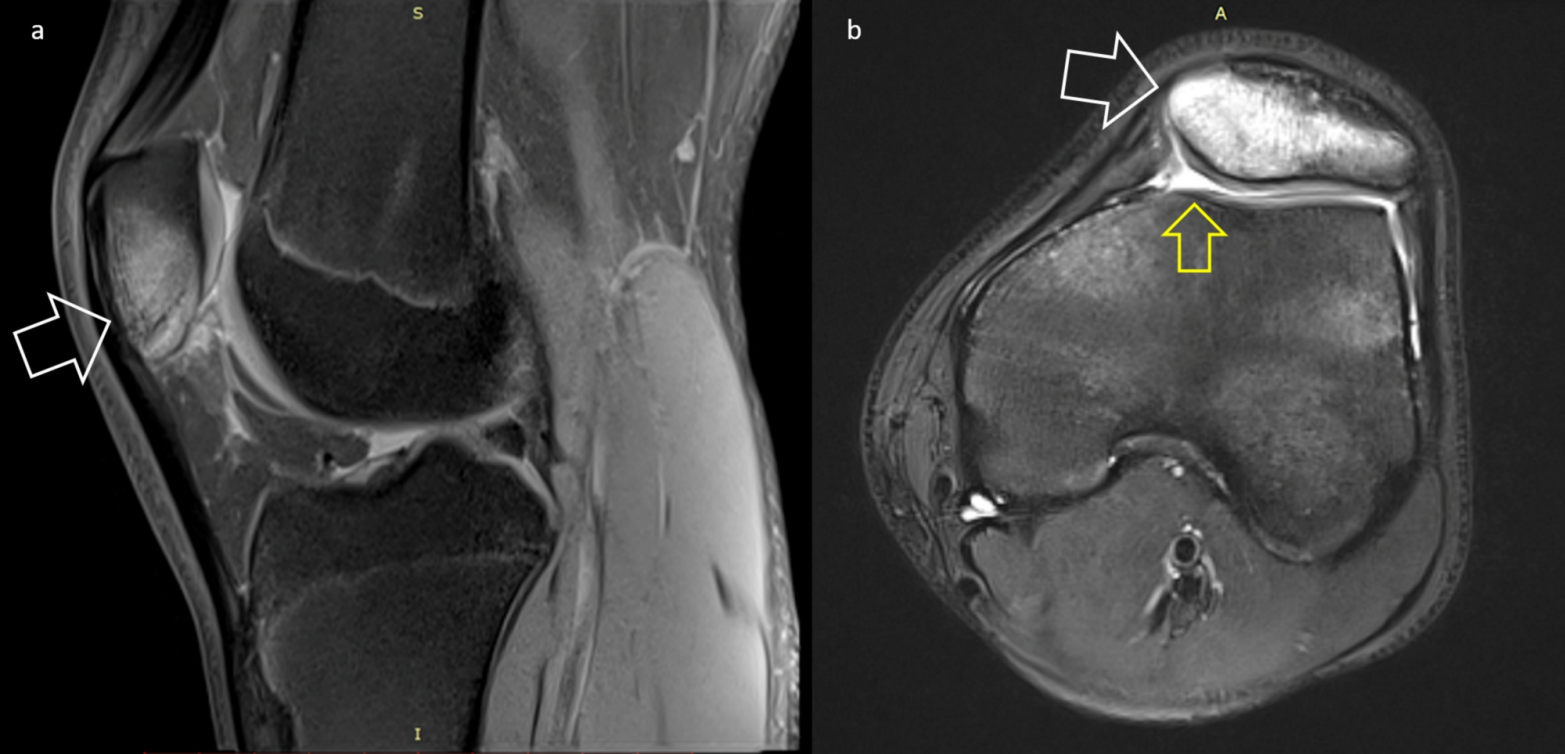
First MRI scan showing diffuse signal uptake of the patella and effusion a. Sagittal view, b. Transverse view White arrows: diffuse signal uptake, Yellow arrow: knee effusion
